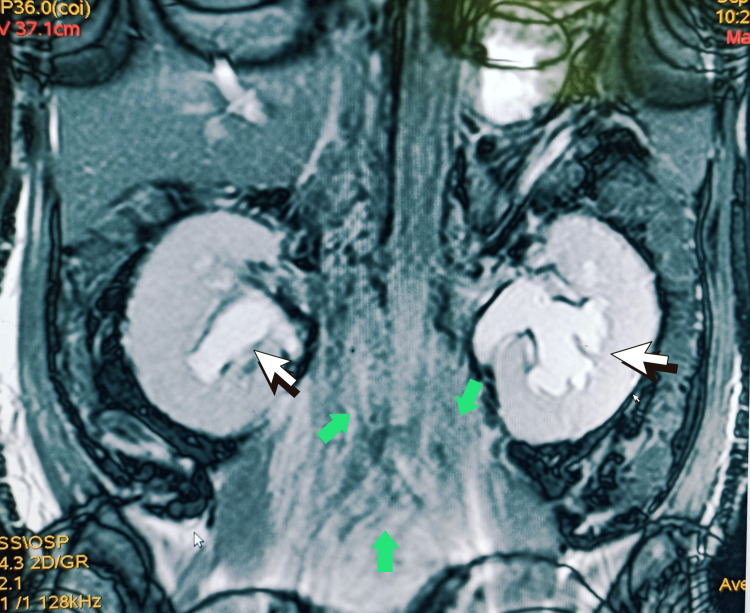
T2W image showing heterogenous soft tissue encasing abdominal aorta extending up to bifurcation (green arrows) Mild fullness of the pelvicalyceal system of bilateral kidneys (white arrows). T2W: T2-weighted image.
